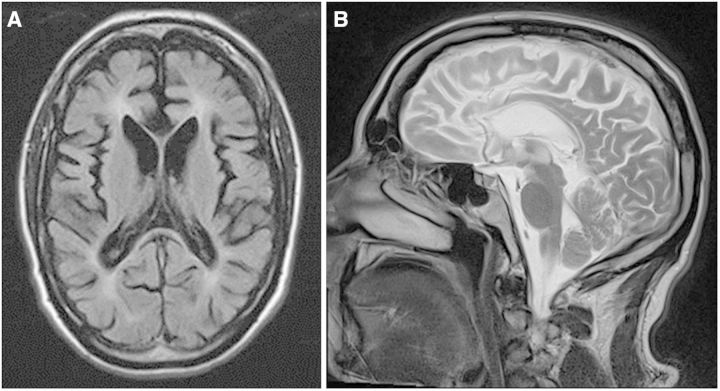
T_1_ axial (A) and T_2_ sagittal (B) MRI in Patient P19. The MRI shows global cerebral atrophy with relative sparing of the occipital lobes and cerebellum, and marked atrophy of the corpus callosum previously described in patients with mutations in *ZFYVE26 *(SPG15) (Goizet *et al.*, 2009).
